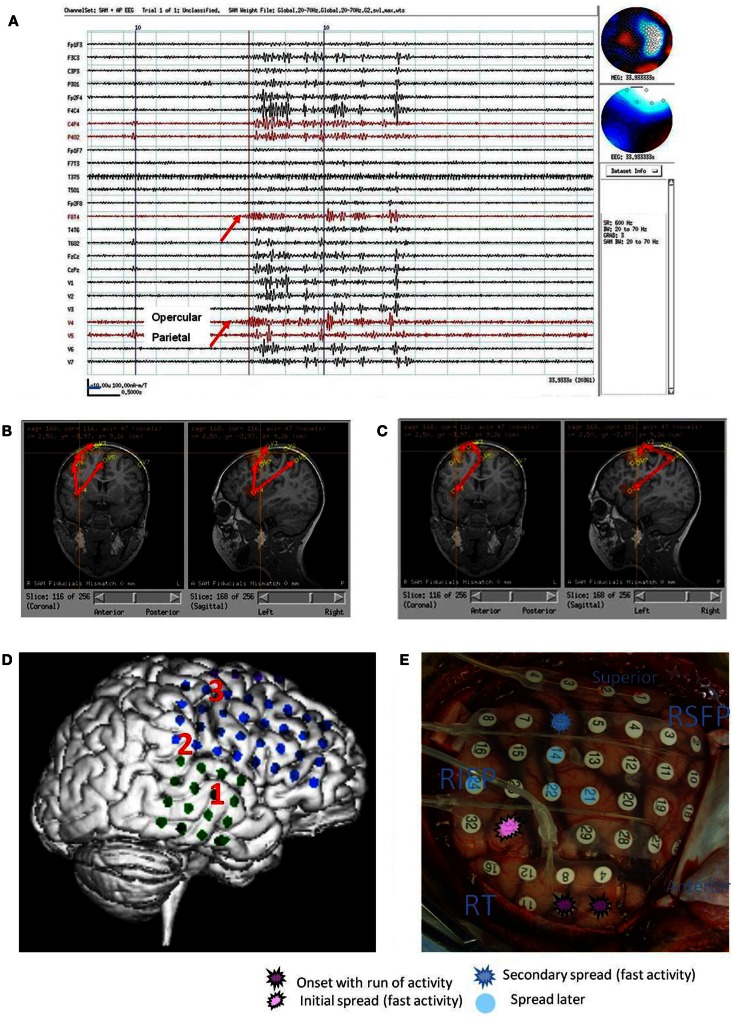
Subject 2. (A) Bipolar AP EEG and seven virtual sensor channels showing onset of burst in unaveraged signals filtered in bandwidth 20–70 Hz, digitized at 600 Hz. Red arrow at V4, right operculum/insula, shows burst onset preceding first apparent onset in F_8_T_4_ EEG channel by 100 ms. For VS locations, onset in location V4 occurred first followed by right mesial parietal V5 (80 ms), then right mid frontal V7 (240 ms), and right superior mid frontal V3 and V1 (260 ms), and finally right posterior frontal V2 (300 ms). (B) Subject’s MRI scan showing one possible pattern of spread based on the timing of the VS peaks. (C) Subject’s MRI showing a second timing pattern that occurred in other bursts (not shown) with earliest onset in superior mesial parietal V5, then superior posterior frontal V3 and operculum/insula V4, and then lateral superior midfrontal V1. Red arrows indicate relative timing of peaks. (D) Subject’s 3D MRI scan and grid electrode locations. Red numbers 1–3 indicate the ictal onset (1) and spread pattern based on ECoG (2, 3). (E) Intraoperative photograph of brain and subdural grids with ictal onset right mid temporal (dark purple), spread to inferior parietal (pink), then superior posterior frontal (dark blue).
